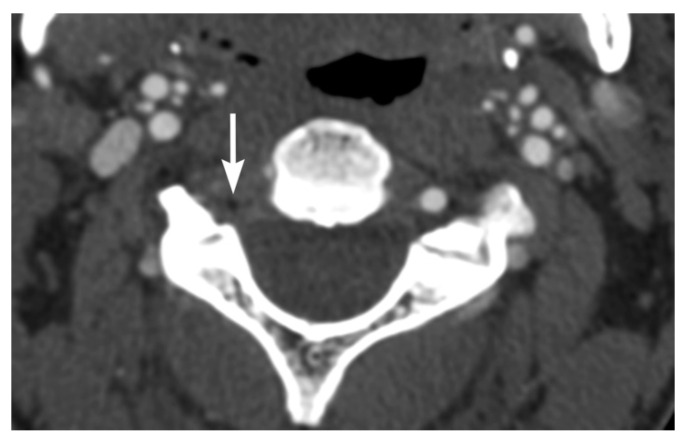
43-year-old male who presented following motor vehicle accident with multiple vertebral fractures and right vertebral artery injury. Axial multidetector CT angiographic image shows occlusion of the right vertebral artery.
